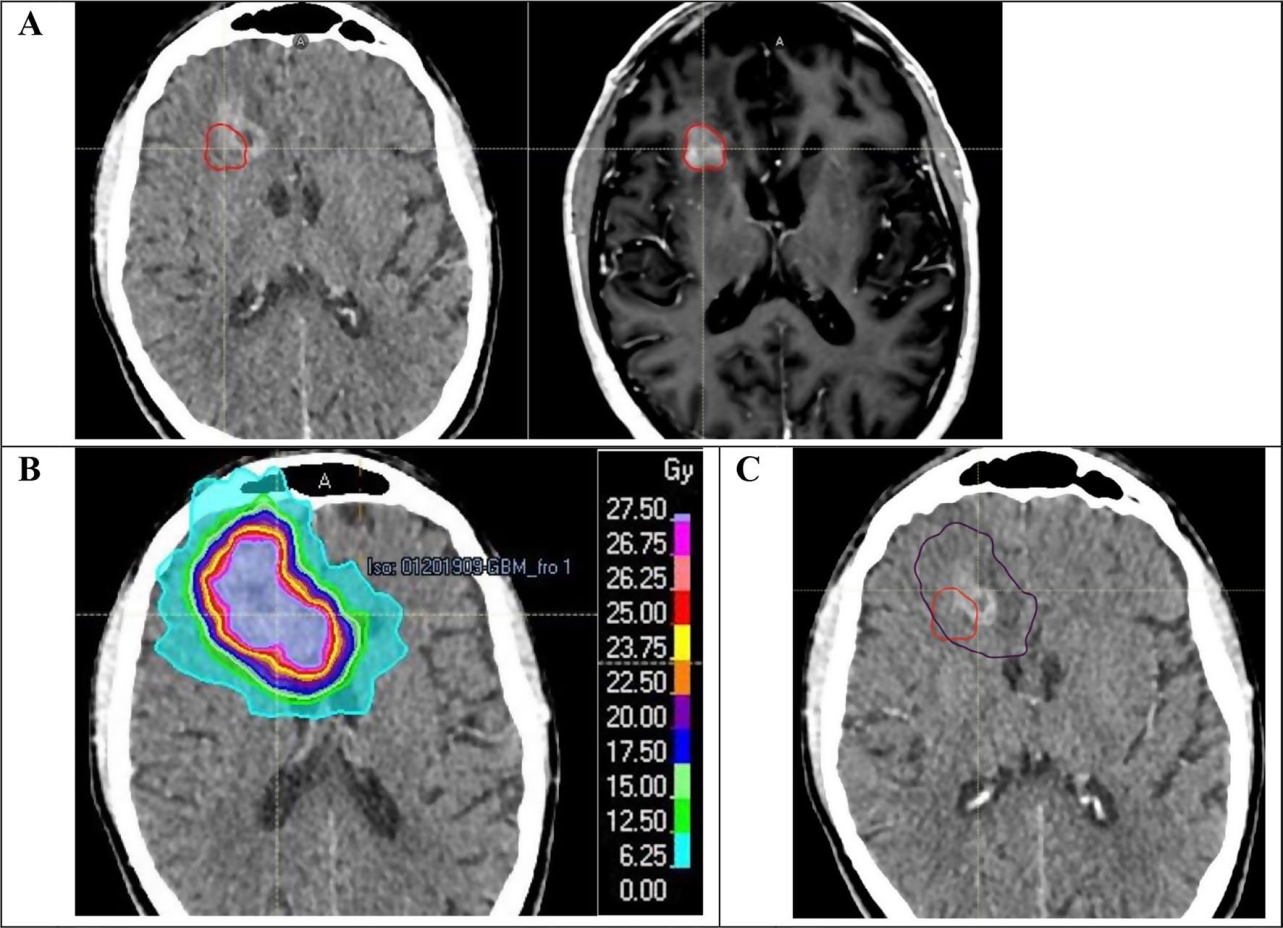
A. MRI showing post-fSRT (delineated in red) recurrence on the right, matched with the fSRT treatment planning scan on the left; B. fSRT treatment plan; C. recurrence post-fSRT (red) and volume which received at least 20 Gy (purple) on the fSRT treatment planning scan
